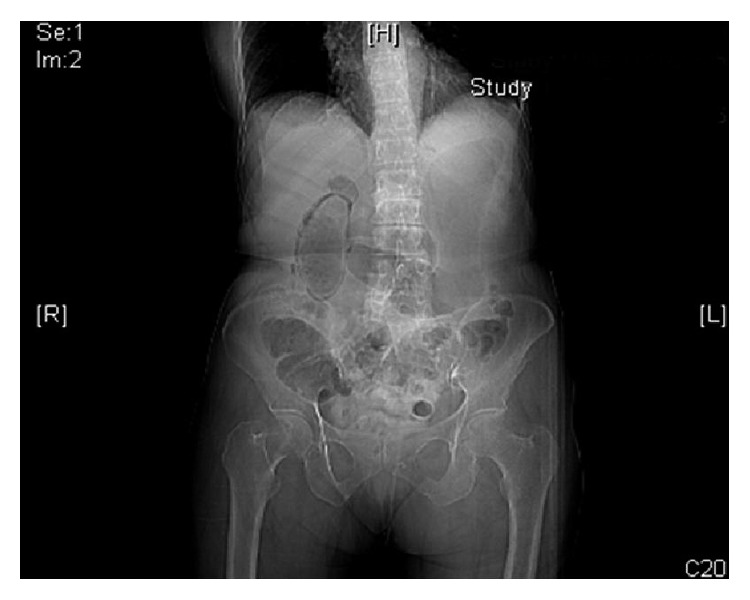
Plain abdominal radiograph. Air outlining the gallbladder.
